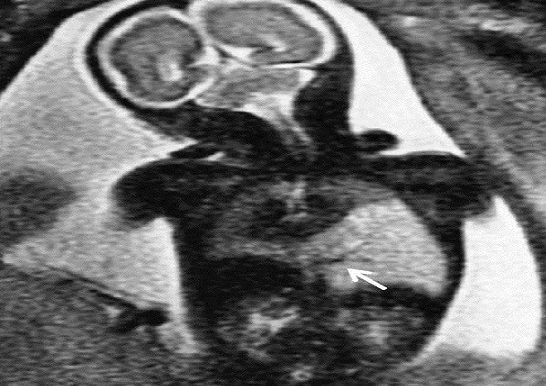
Coronal prenatal MR image: left-sided triangular pulmonary mass of increased signal intensity. Note systemic feeding artery arising from the abdominal aorta (arraw)
